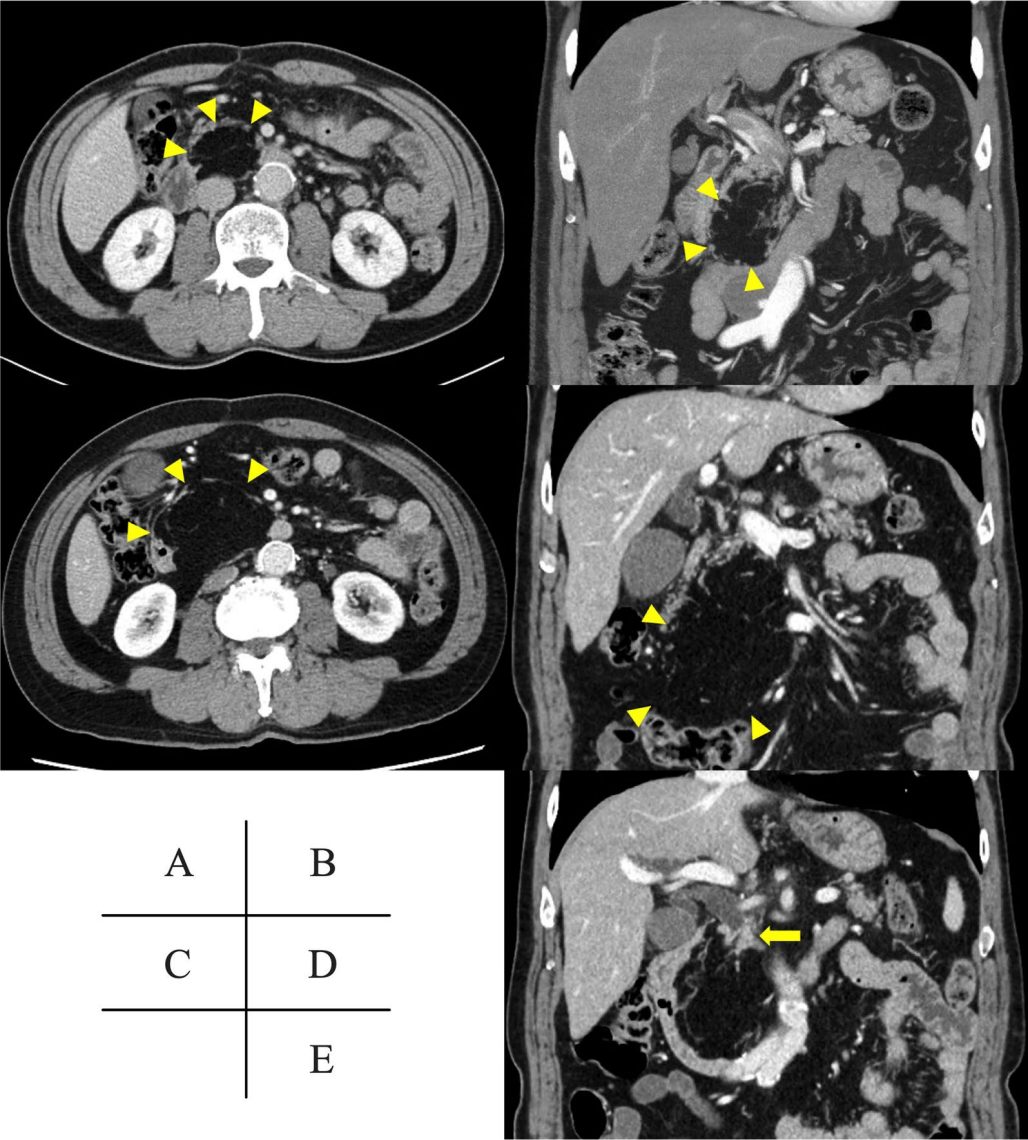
A, B Contrast-enhanced CT performed when the patient was diagnosed with pancreatic lipoma 12 years previously. A well-defined 6 cm mass composed of homogeneous adipose tissue without any solid nodules in the pancreatic head is visible (arrowheads). C–E Contrast-enhanced CT before surgery. The previously diagnosed 12 cm adipose tumor is visible in the pancreatic head (arrowheads). An irregular isodense mass appeared at the upper margin of the tumor (arrow), which invaded and obstructed the distal common bile duct
